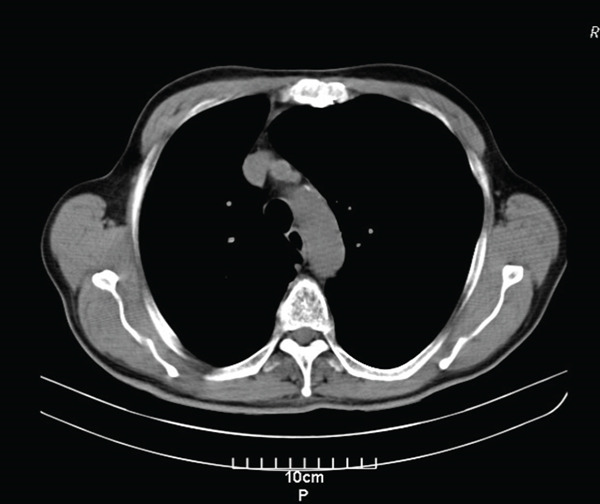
Chest CT scan, mediastinal view, hyperinflation of the left lung and the normal position of the aorta that rules out dextrocardia.
